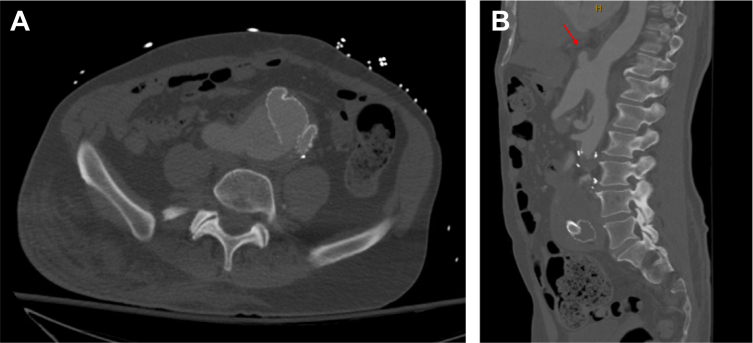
Computed tomography images demonstrating (A) a pseudoaneurysm arising from the right iliac limb in the axial plane and (B) a pseudoaneurysm arising from the supraceliac anastomosis (*red arrow*) in the coronal plane.
